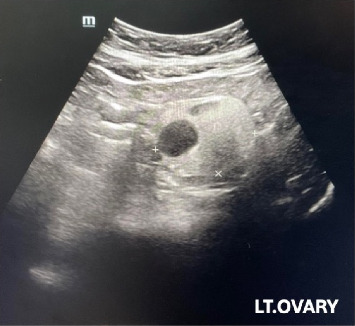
Ultrasound of the abdomen showing a 5 cm hyperechoic lesion with an internal cystic component in the left ovary.
